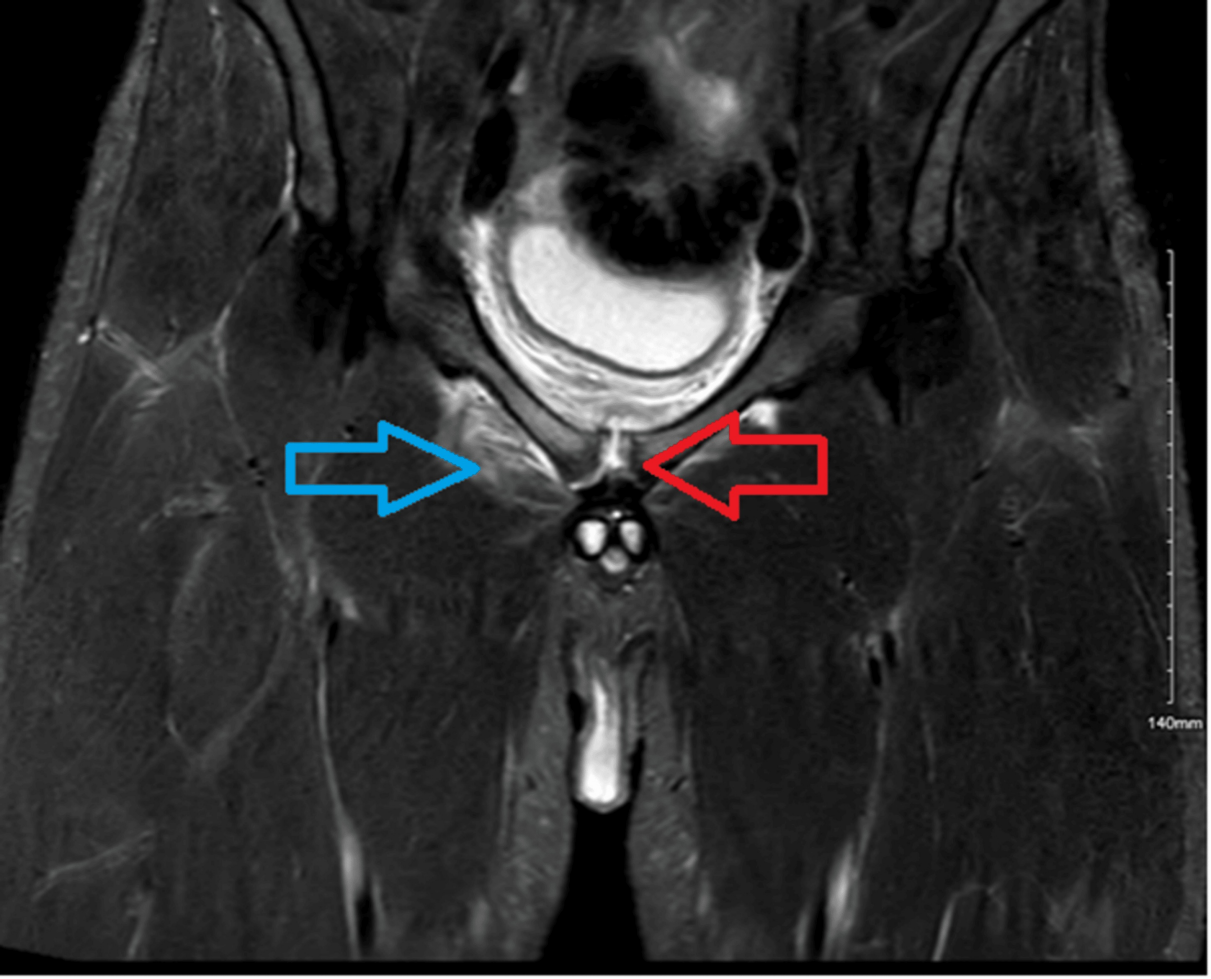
MRI scan Small foci of septic fluid are visible in the vicinity of the pubic symphysis. The blue arrow denotes edema and collection in the right obturator internus, whereas the red arrow shows foci in the pubic symphysis.
